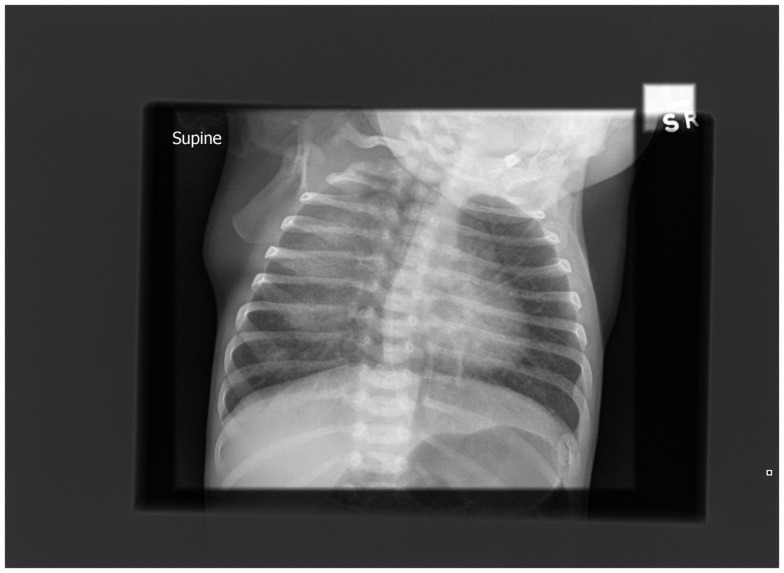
Chest radiograph on admission showing right-sided changes.
